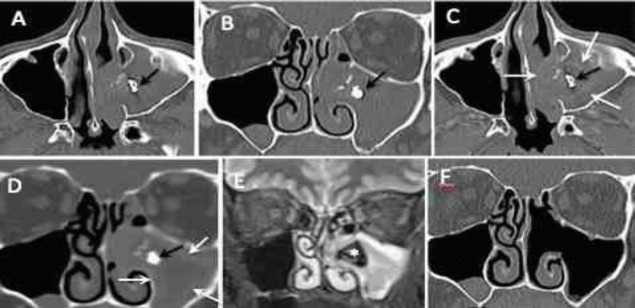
Axial (A) and coronal (B) non-enhanced computed tomography scans; axial (C) and coronal (D) contrast-enhanced computed tomography scans. Complete opacification of left maxillary sinus, nasal cavity, and ethmoid air cells, showing an iron-like density (black arrow) within the maxillary sinus and microcalcifications. There is an associated expansion of the left maxillary sinus. Mucosal membrane thickening around the fungus ball is visible in contrast-enhanced computed tomography scans (C,D) (white arrows). Coronal T2-weighted magnetic resonance imaging (E) with a very low, inhomogeneous signal due to high protein and low water content (small star). Coronal non-enhanced computed tomography scans (F), showing normal air-filled maxillary sinus after surgery
